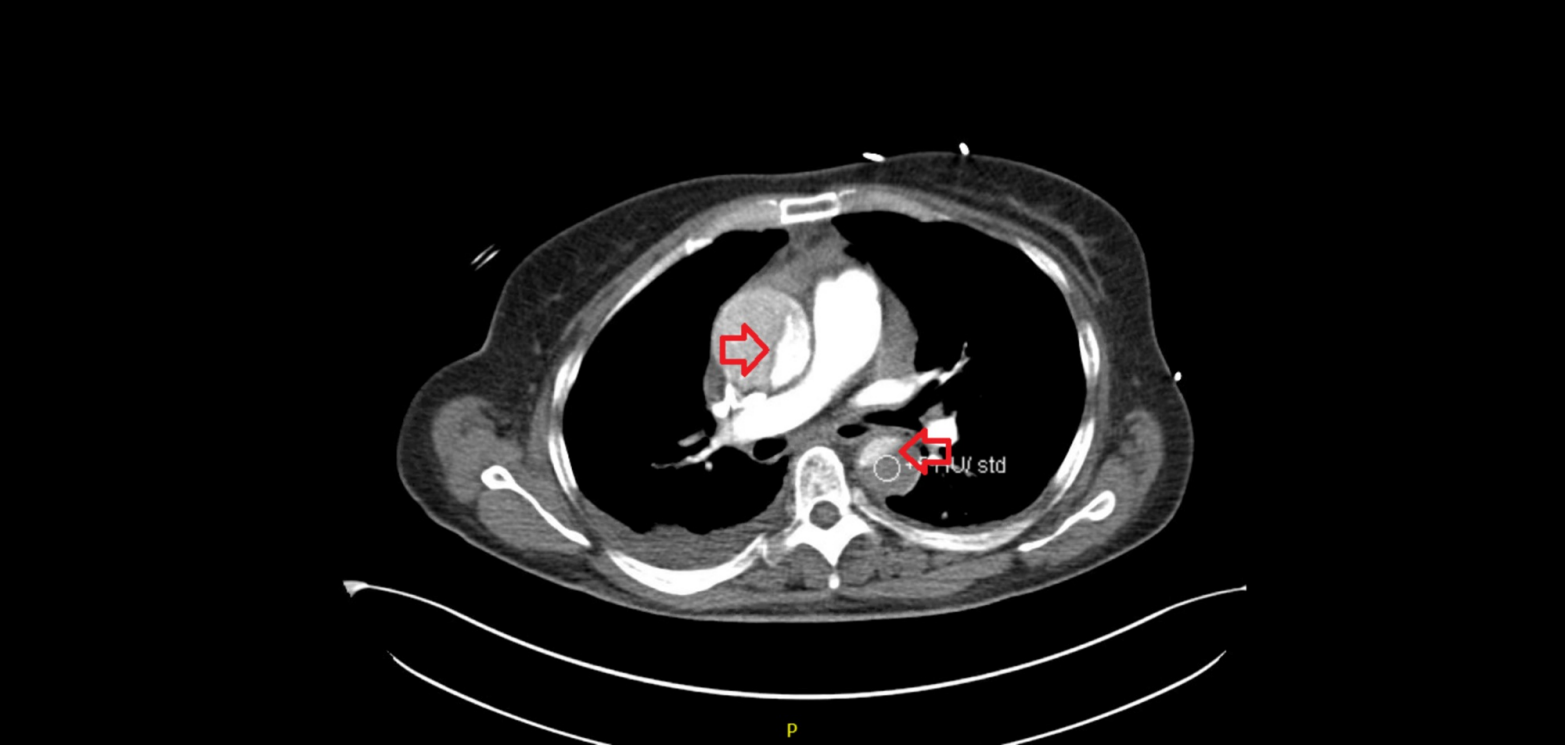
CT angiogram revealing Stanford type A AAD originating from the ascending and extending down the descending aorta
